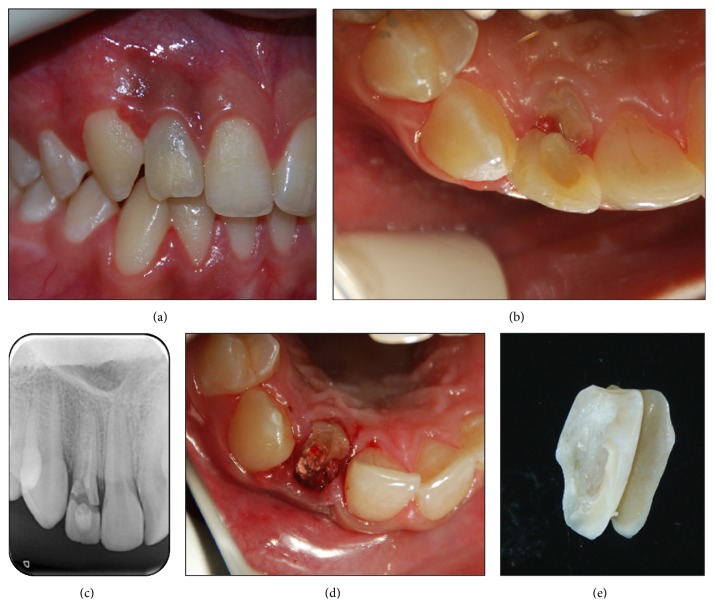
(a) Intraoral buccal view of a crown-root fracture. (b) Intraoral occlusal view of a crown-root fracture. (c) Preoperative intraoral radiograph showing the extension of the fracture. (d) View of the remaining root fragment after the removal of the coronal fragment. (e) View of the extracted coronal fragment.
